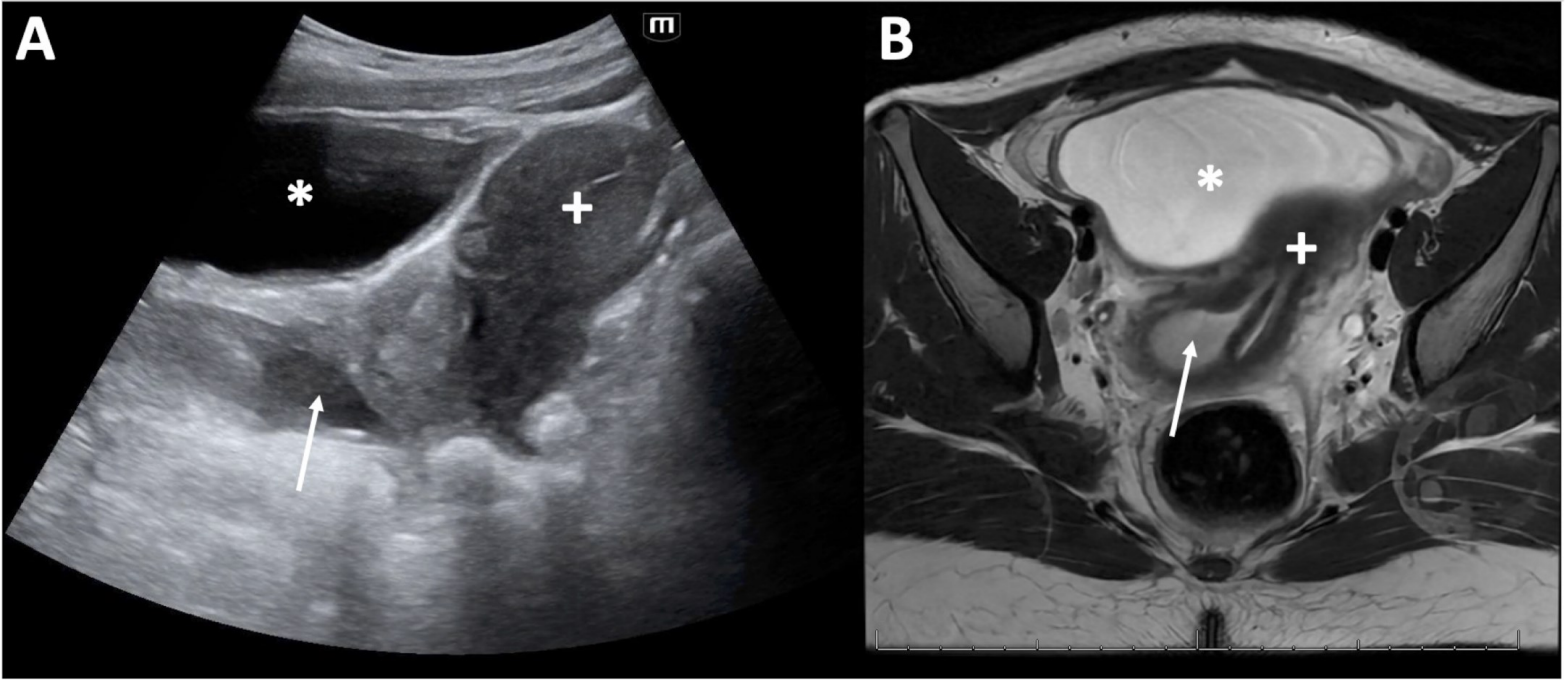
Bladder (asterisk), uterus (plus sign), andhematocolpos in right side of septate vagina (arrow) initially visualized on point of care ultrasound (A) and redemonstrated on MRI (B).
